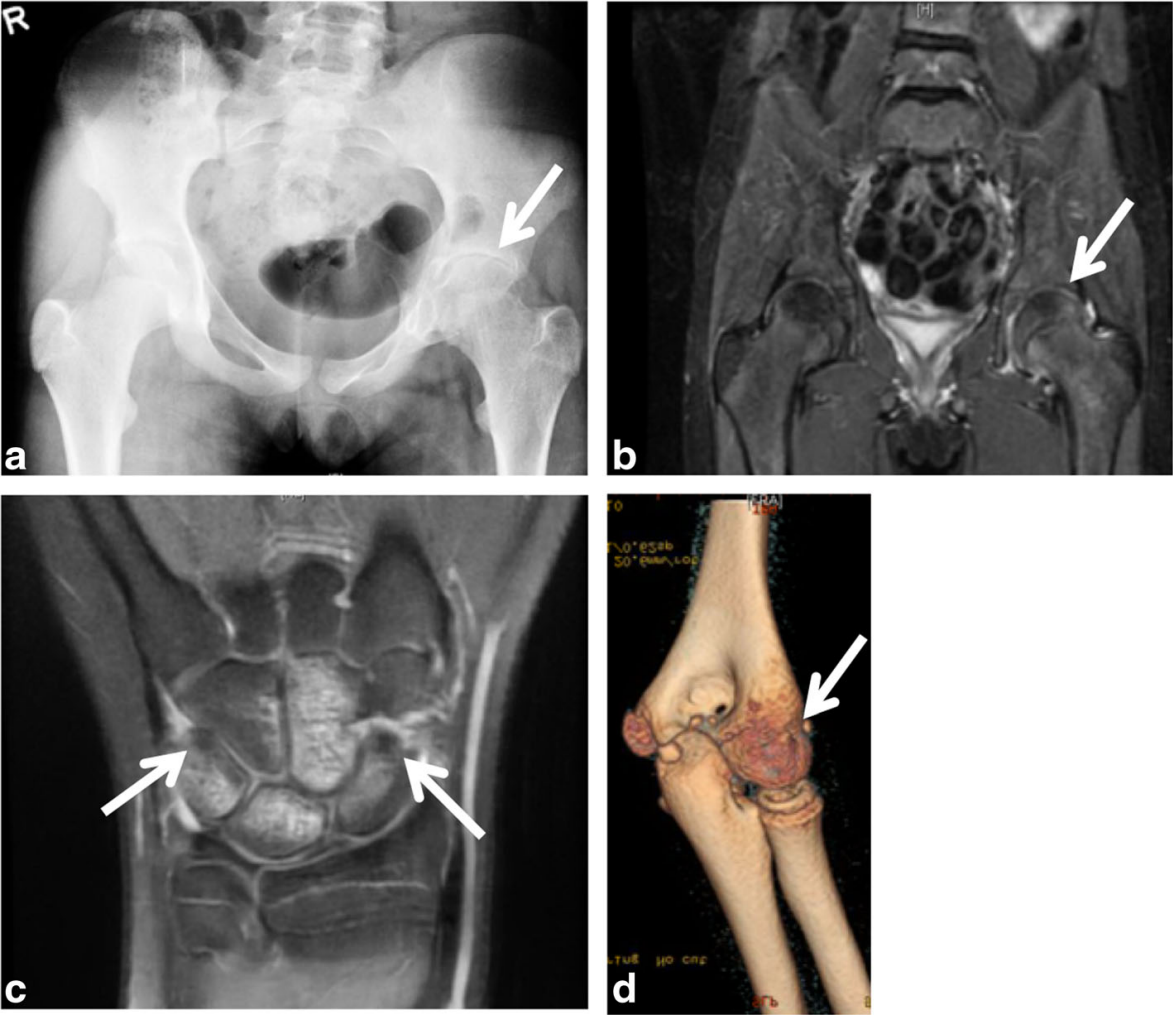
Representative images of severe monoarthritis in children diagnosed with oligoarticular juvenile idiopathic arthritis. a X-ray of the hips showed shortening of the left femoral neck and decreased joint space; b MRI with gadolinium revealed enhancement and mild thickening of the left hip synovium on T2-weighted imaging; c MRI of the right wrist demonstrated carpal synovitis, marked bone marrow edema, bone cysts and erosions on T1-weighted imaging; d Non-contrast CT of the left elbow showed bone erosions, hyperostosis of the trochlear-olecranon complex and reduced joint space. *White arrows* point towards the abnormal findings
